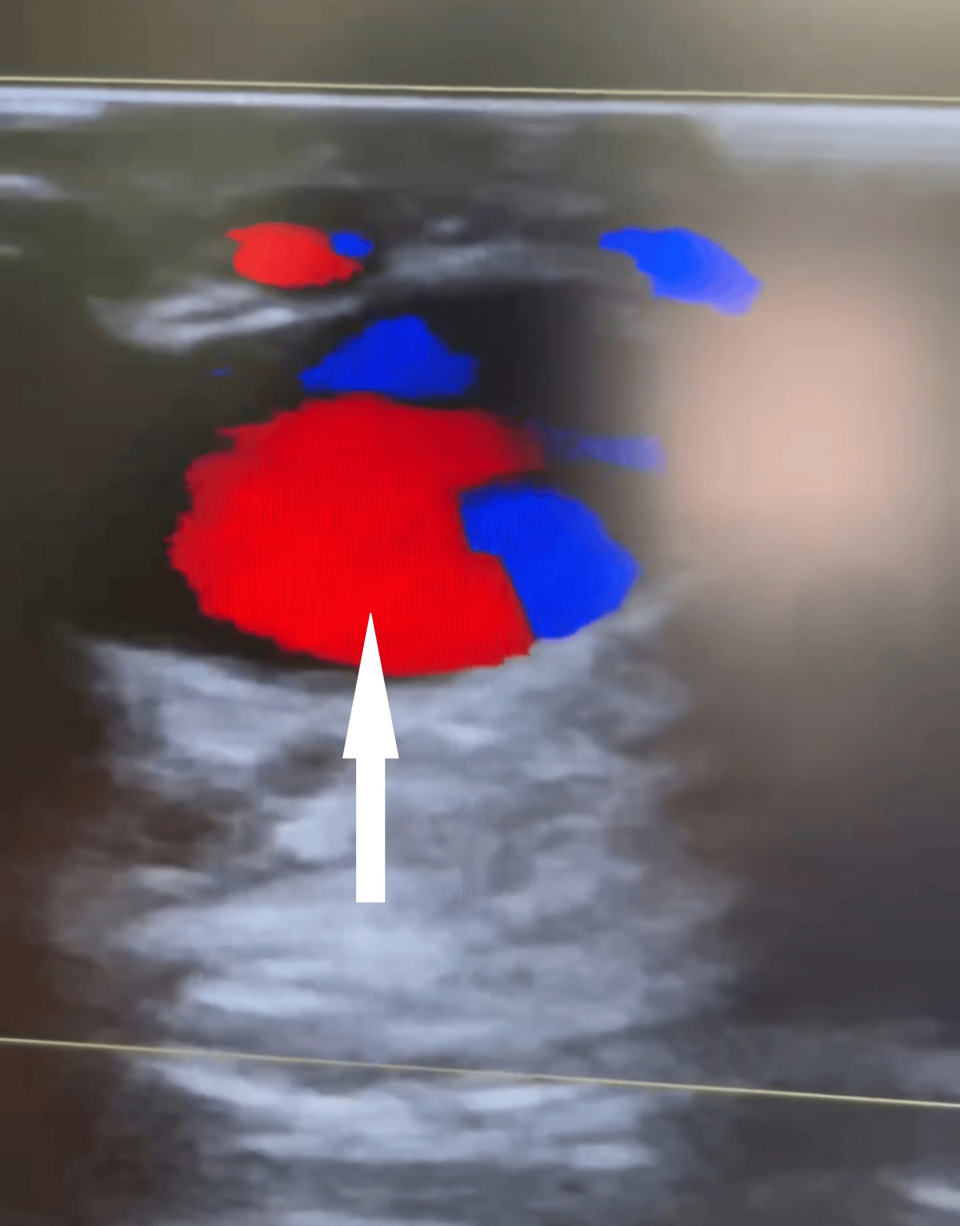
Color Doppler ultrasound demonstrating the presence of a facial artery pseudoaneurysm in the right buccal region, with clear visualization of turbulent blood flow within the lesion
